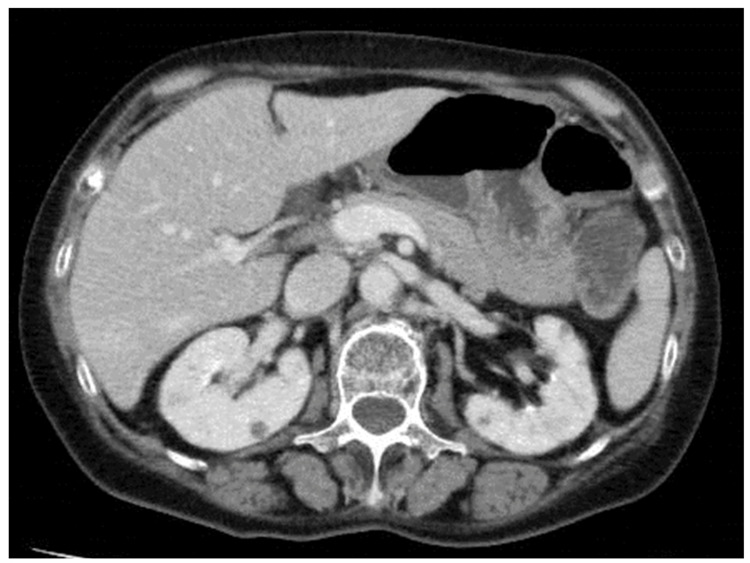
Computed tomography of the shrinking lesion in the abdomen seven months after the initiation of encorafenib, binimetinib, and cetuximab treatment.
